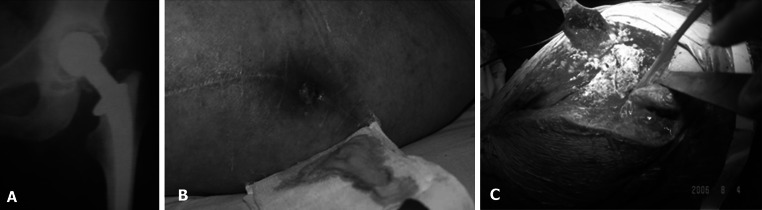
a X-Ray films of THR postoperative infection. b Sinus formation postoperative infection. c Pub substances were observed during operation
